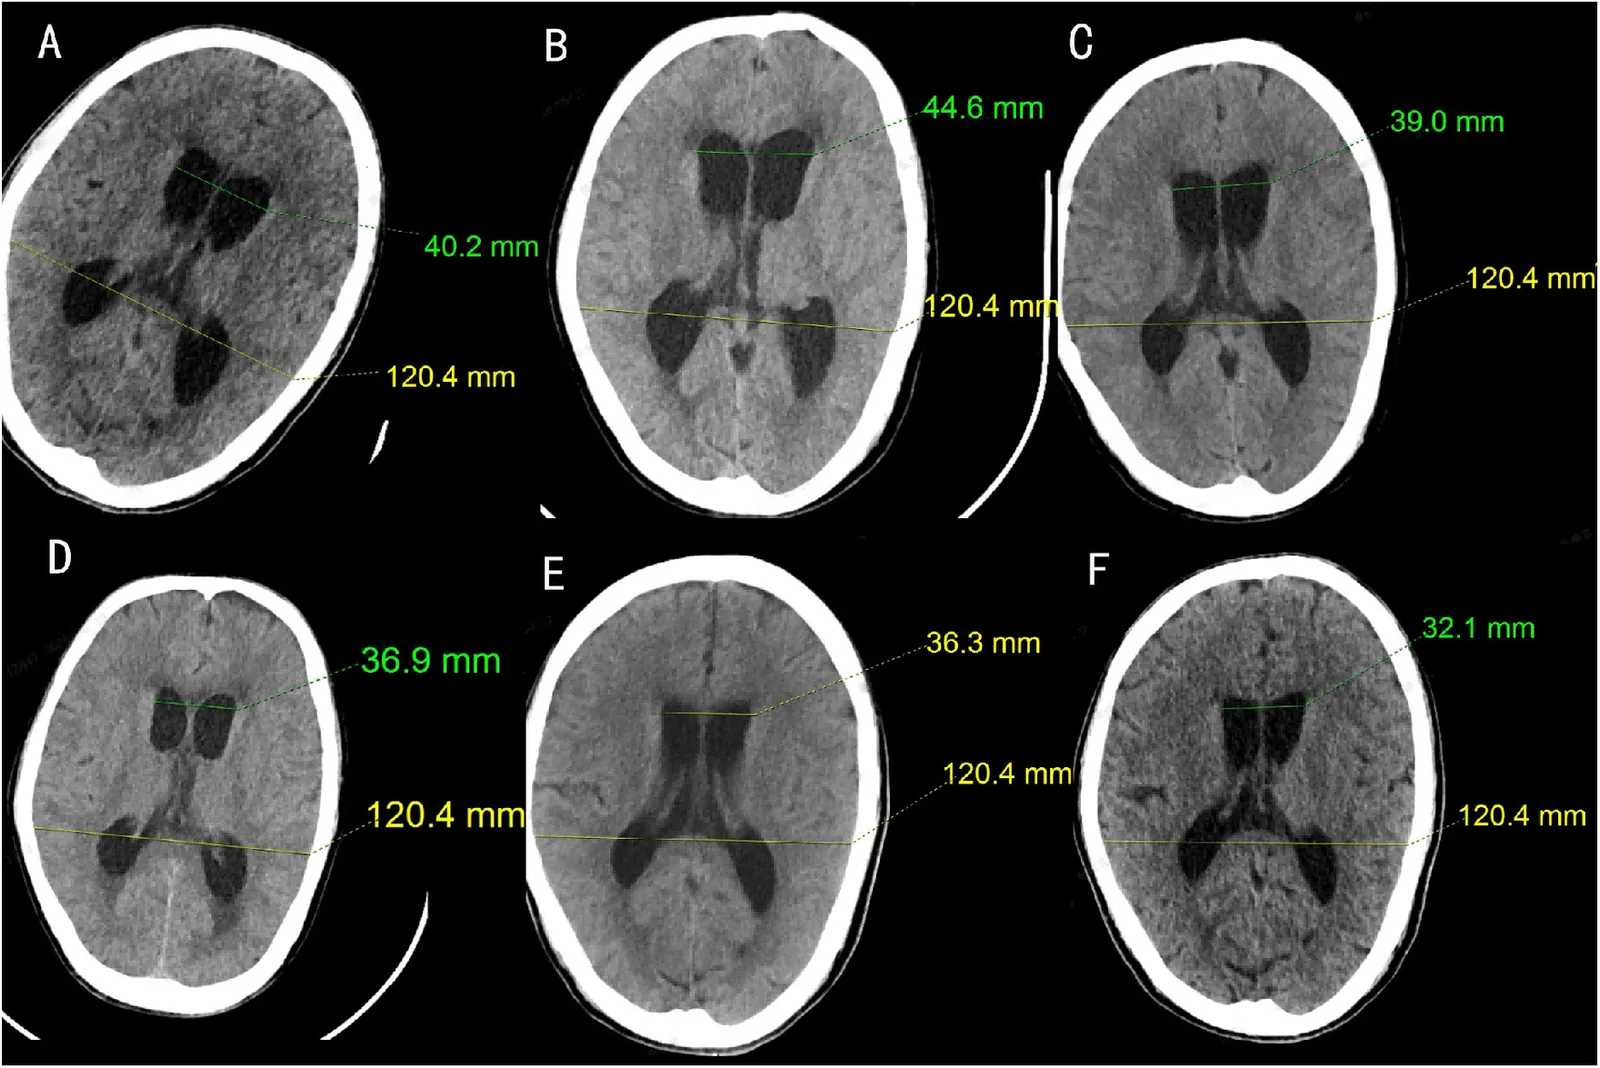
Serial non-contrast cranial CT scans demonstrating the dynamic evolution of hydrocephalus. (A) Hospital day 1: marked supratentorial ventricular dilatation with periventricular hypoattenuation (Evans index = 0.334). (B) Hospital day 12: transient worsening of ventricular dilatation (Evans index = 0.370). (C) Hospital day 19: partial improvement in ventricular size (Evans index = 0.324). (D) Hospital day 26: significant reduction in ventricular dimensions with resolution of periventricular hypoattenuation (Evans index = 0.306). (E) 3-month follow-up: near-complete resolution of ventricular dilatation (Evans index = 0.301). (F) 10-month follow-up: complete normalization of ventricular size with no recurrence (Evans index = 0.267). Evans index = maximum width of the frontal horns/maximum inner diameter of the calvaria (normal < 0.30).
